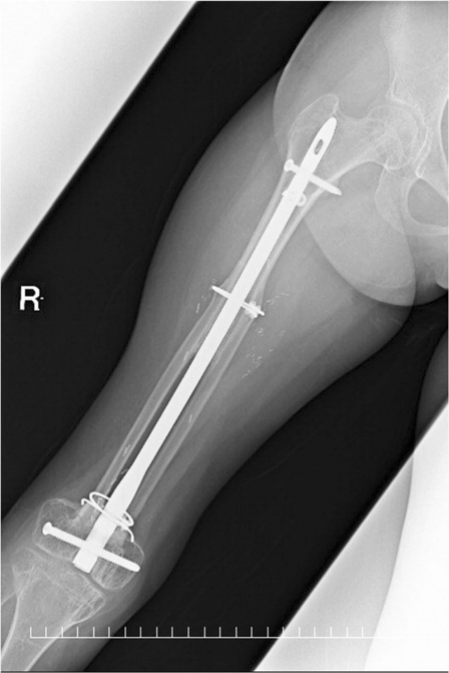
Postoperative X-ray obtained after 20 months shows complete bone union
